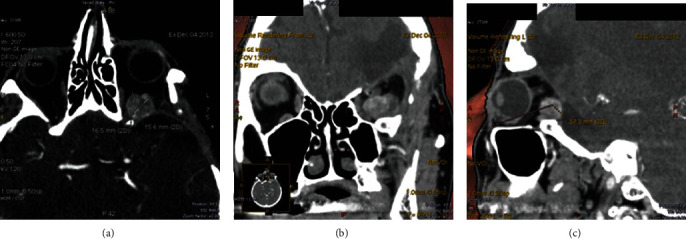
Case 3. (a) CT imaging showing a well-defined rounded lesion, in the left orbital apex. (b) Coronal view. (c) Sagittal view.
